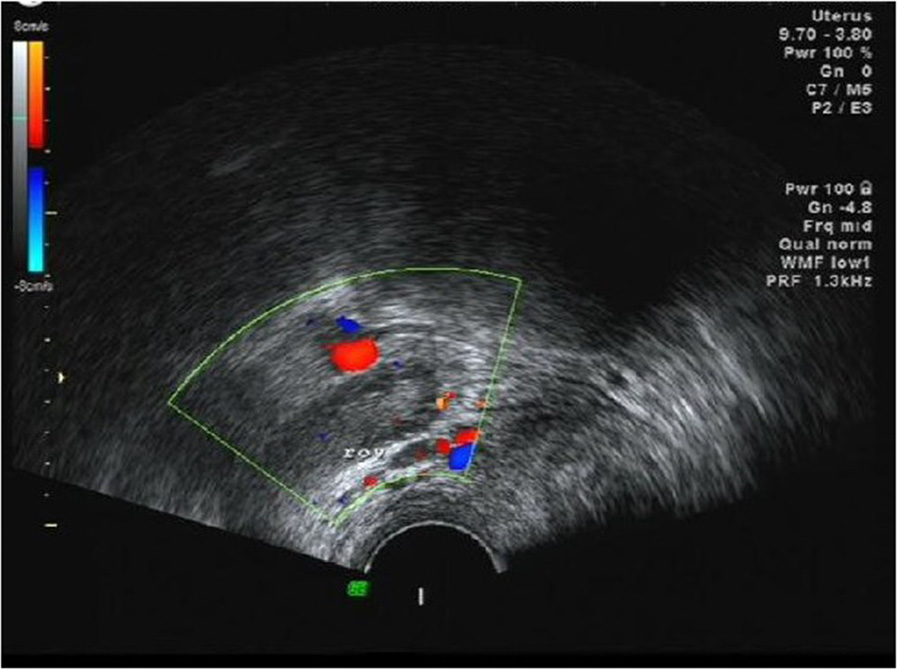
An ultrasound scan (on 13 December 2013) showed a right adnexal mass and nothing abnormal was detected in the left attachment area.
